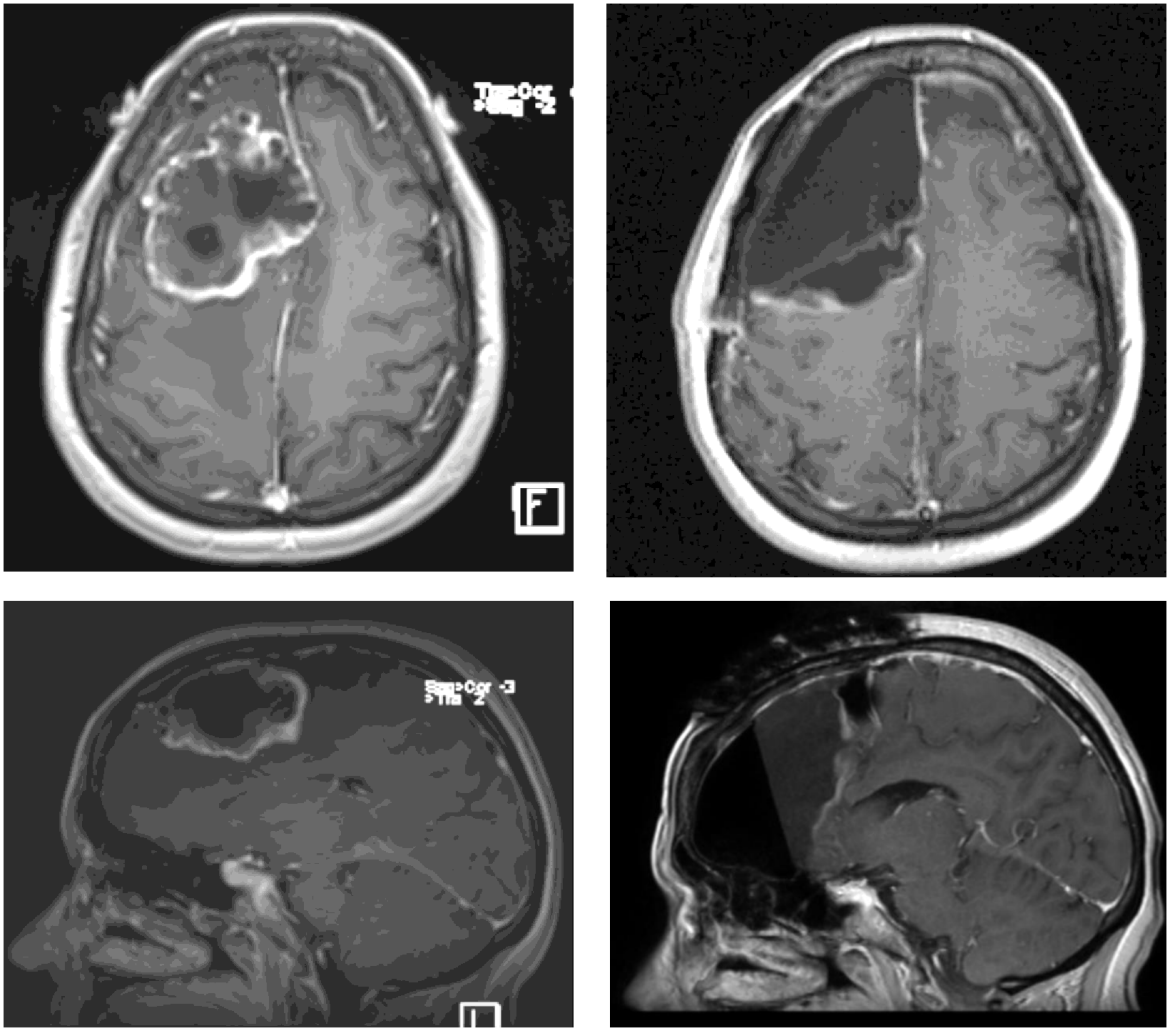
Pre and post-operative axial and sagittal T1-Weighted post contrast MRI of large right frontal BM treated with gross total resection (GTR).
